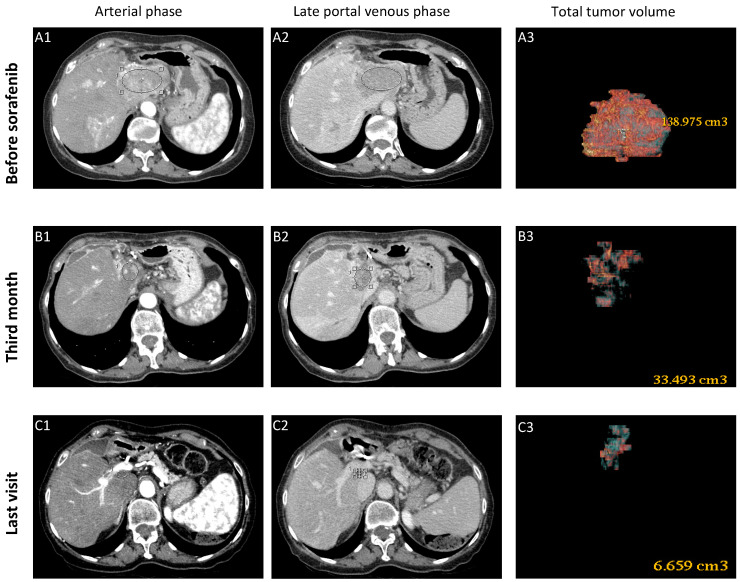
Baseline CT scan (A1,A2) showing the large HCC mass (maximum diameter 75 mm) in the left liver lobe with contrast enhancement in the arterial phase and wash-out in the late portal phase with vascular invasion. Follow-up CT scans demonstrated the markedly reduced sized necrotic lesion (maximum diameter 32 mm) at the third month of therapy (B1,B2) that remains barely noticeable (maximum diameter 18 mm) at the last visit (C1,C2), with no contrast enhancement in both phases. Total tumor volume (TTV) declined from 138.9 cm^3^ (A3) to 33.4 cm^3^ (B3) in 3 months, and to 6.6 cm^3^ at the last visit (C3) after 48 months from the beginning of therapy.
